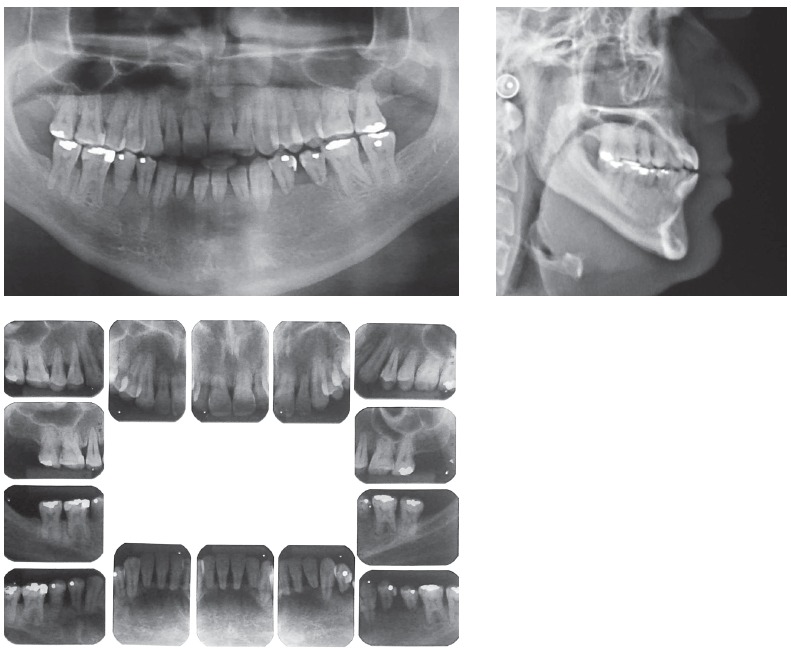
Baseline panoramic, periapical and lateral radiographs.
